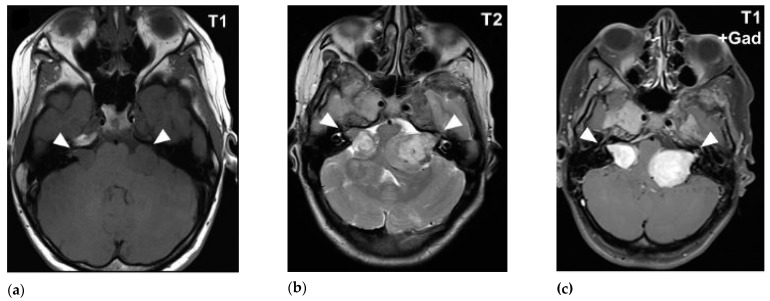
MRI imaging of bilateral NF2 VS in cerebellopontine angle: (a) T1-weighted MRI reveals bilateral NF2 VS as hypo-intense signals; (b) T2-weighted MRI and (c) T1-weighted MRI with contrast demonstrates hyperintensity of bilateral NF2 VS [53].
